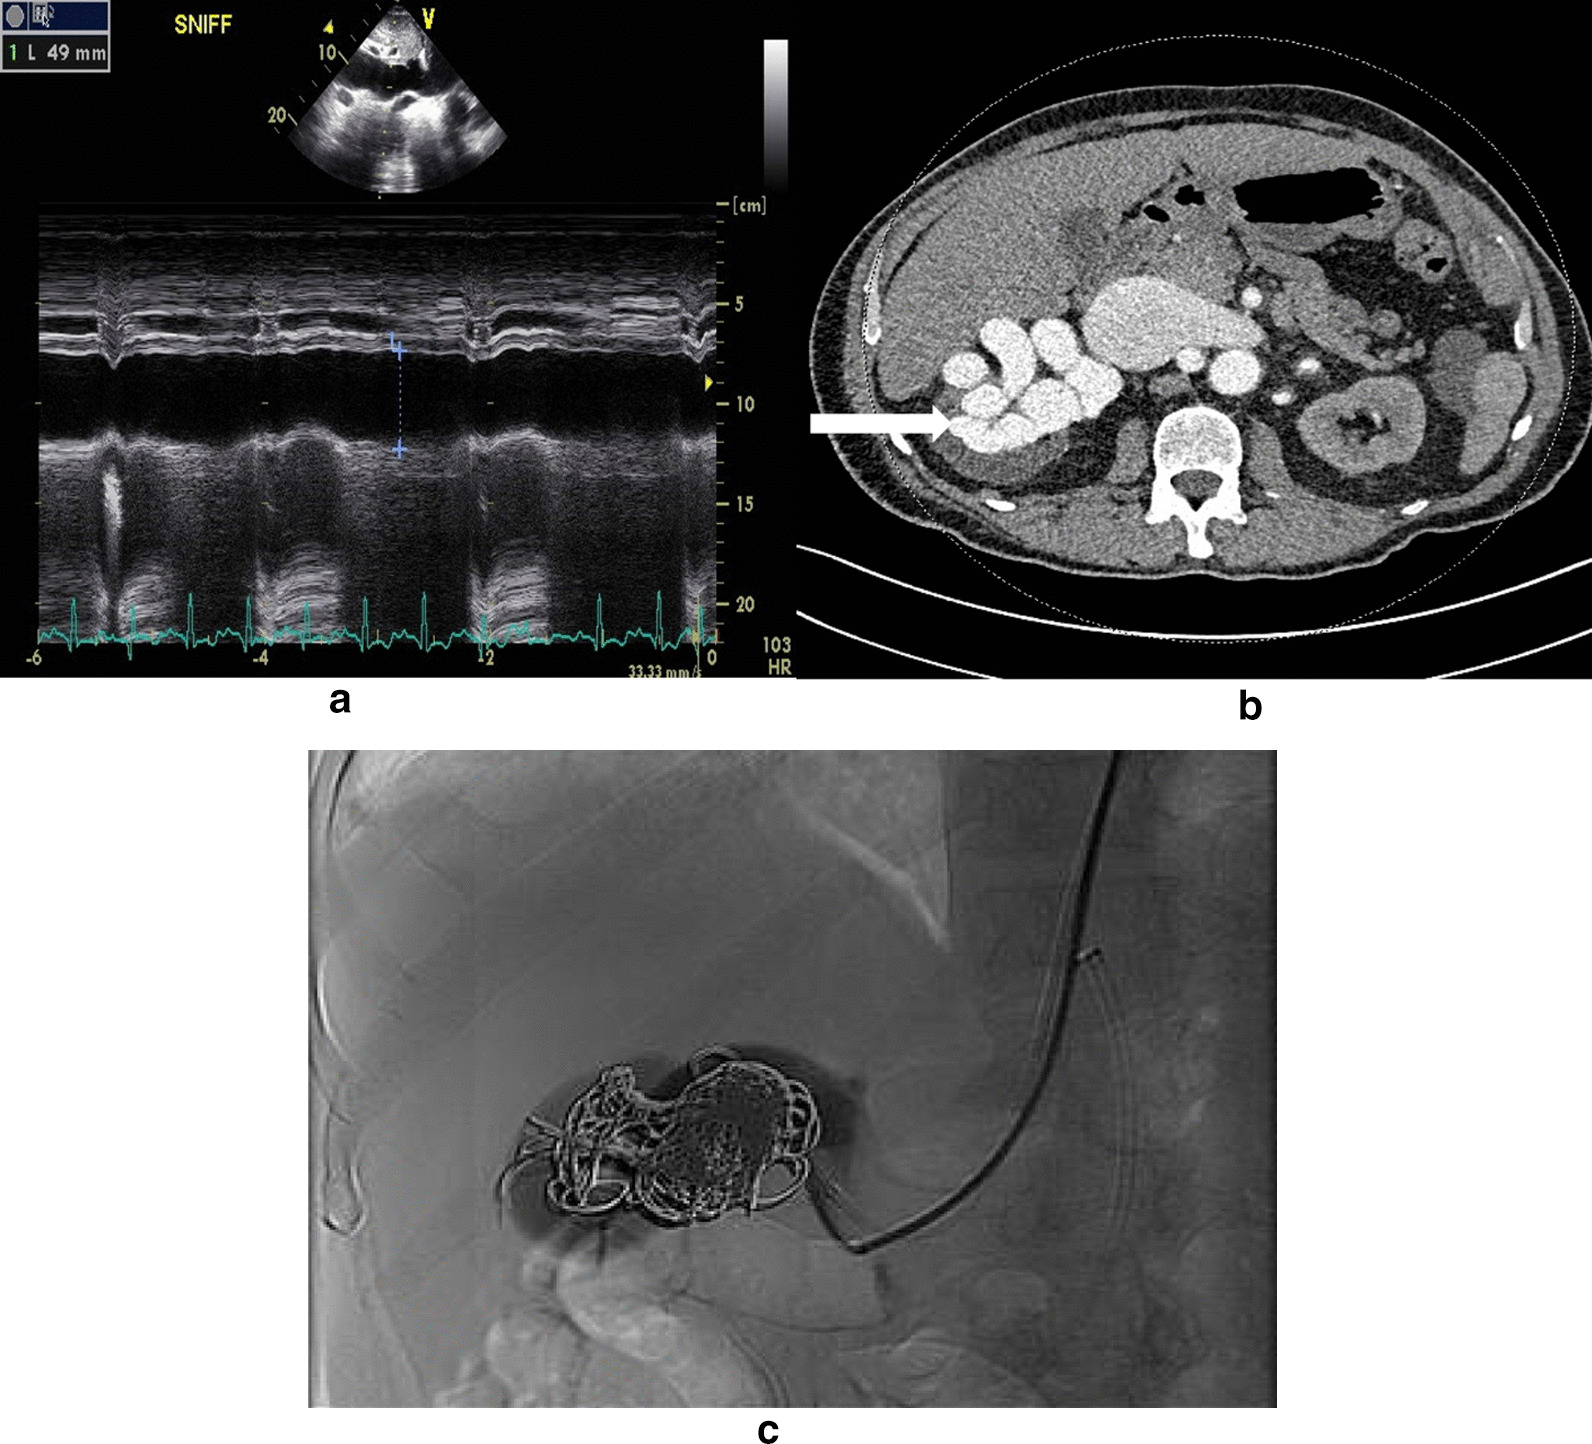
Still frame echo image of distended inferior vena cava (a), computed tomography of the right renal arteriovenous malformation delineated with contrast, marked by the arrow (b), still frame post-coil embolization catheterization (c)
